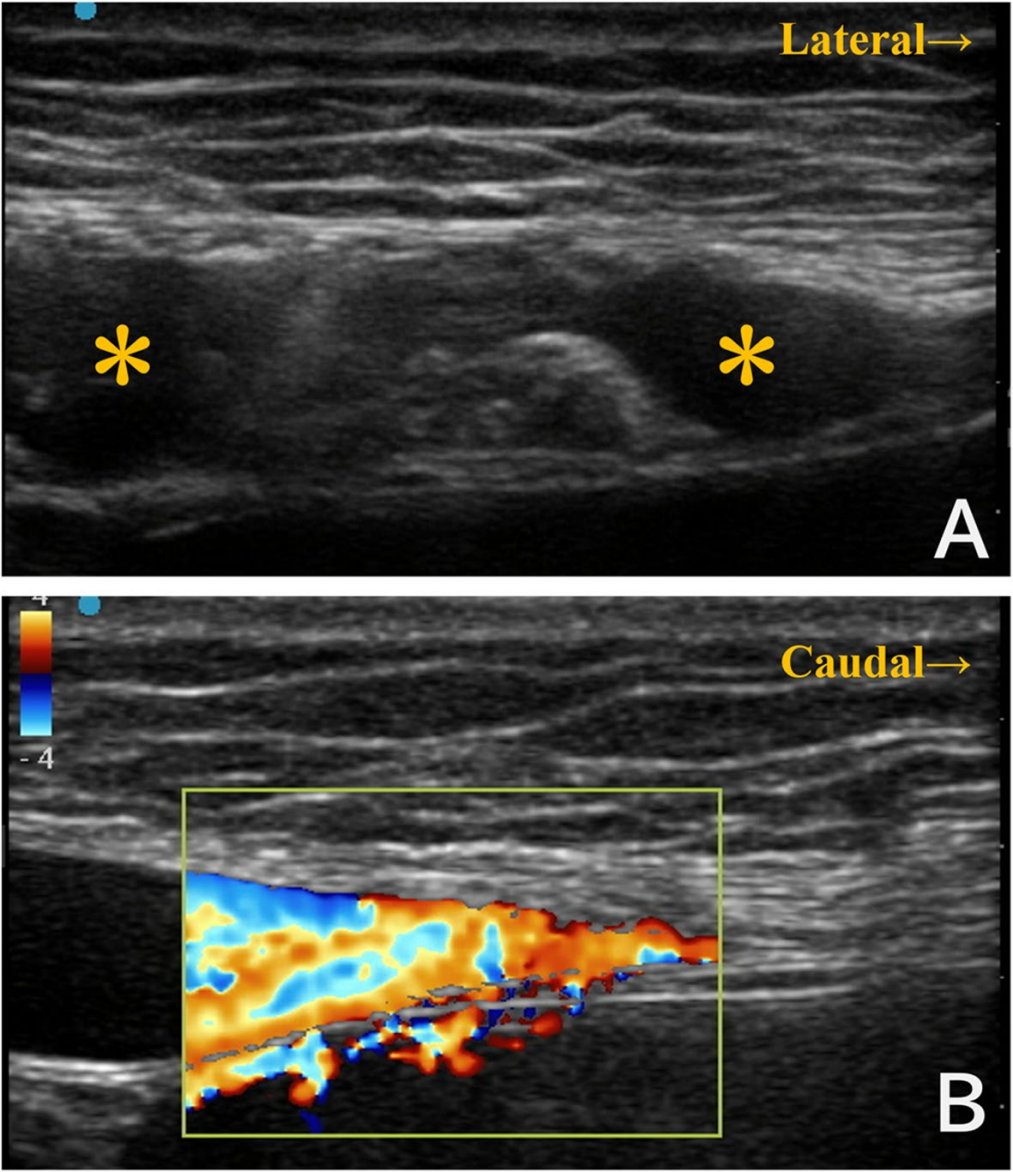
Ultrasound findings during attempted rectus sheath block. (A) Axial ultrasound image showing vascular structures (＊) within the left rectus abdominis muscle. (B) Sagittal Doppler ultrasound confirmed vascular flow
